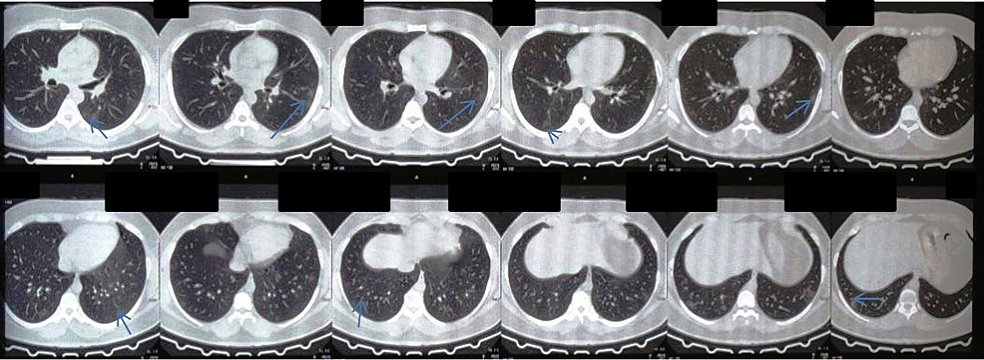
HRCT thorax finding of the patient showing typical changes of GGO GGO are marked with blue arrows. HRCT: high-resolution computed tomography; GGO: ground-glass opacity
